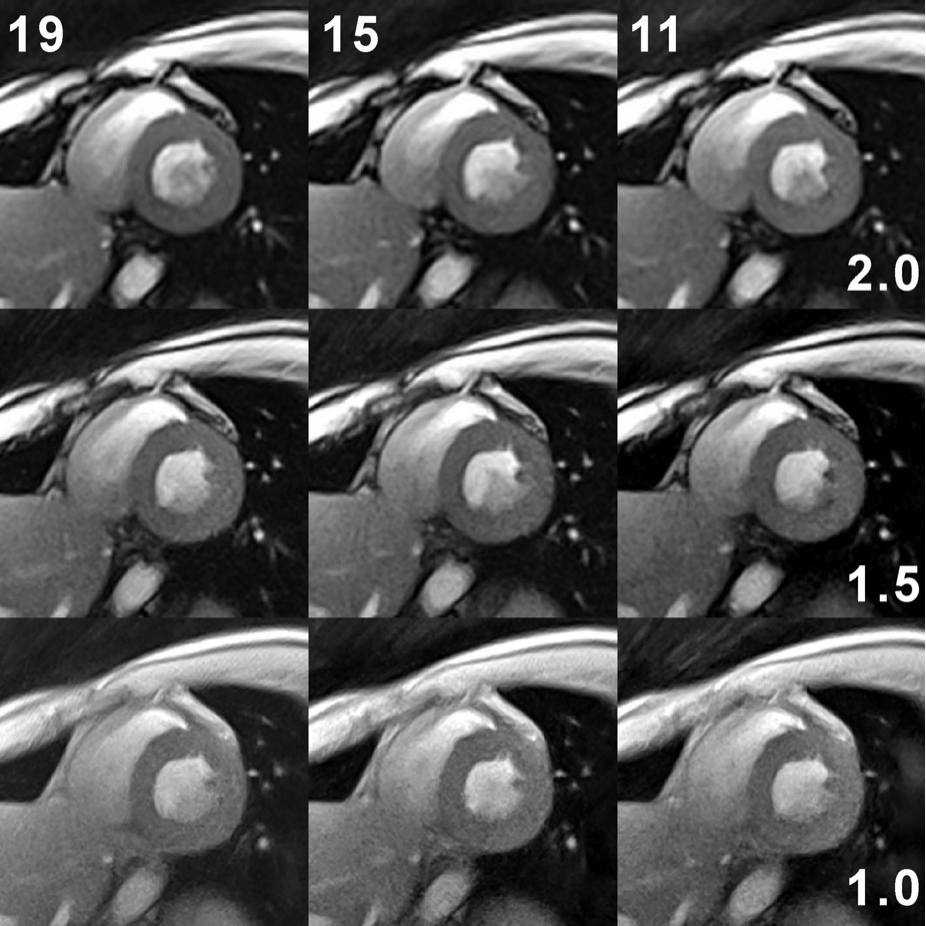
Spatial vs temporal resolution in real-time radial FLASH CMR. T1-weighted images (spoiled FLASH, flip angle 8°) of a short-axis view during systole at 2.0 mm (TR/TE = 2.0/1.3 ms), 1.5 mm (TR/TE = 2.2/1.4 ms), and 1.0 mm resolution (TR/TE = 2.8/1.8 ms) using data sets with only 19, 15, and 11 spokes, respectively. Individual acquisition times are given by the # spokes × TR and range from 22 ms (upper right: 11 spokes and TR = 2.0 ms) to 52 ms (lower left: 19 spokes and TR = 2.8 ms). The images were selected from respective real-time CMR movies reconstructed by nonlinear inversion and zoomed by a factor of 1.5.
